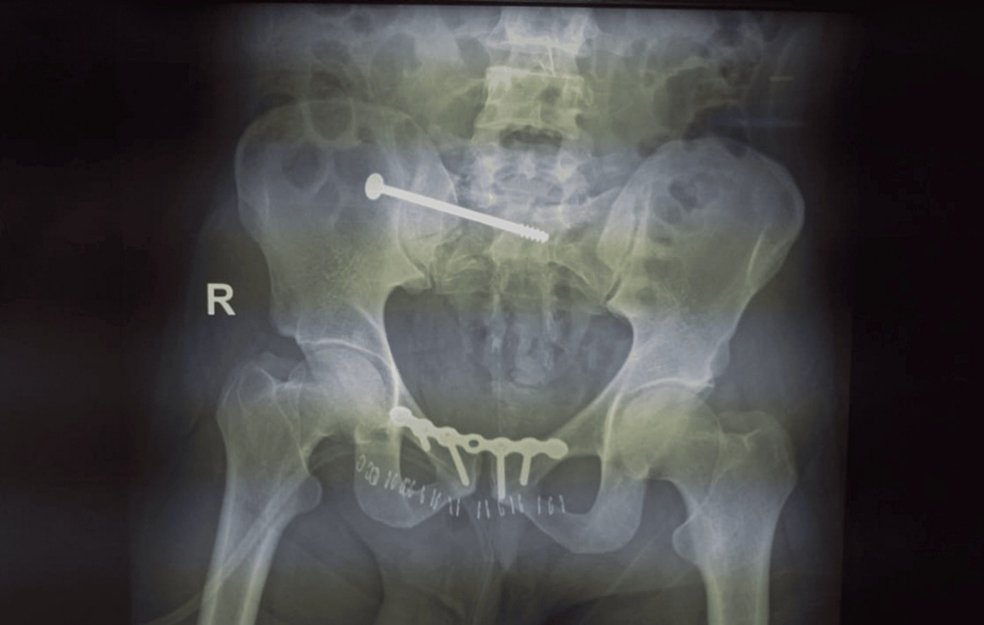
Postoperative X-ray posteroanterior view of the pelvis showing excellent reduction and fixation of the sacral fracture using an iliosacral screw with fixation of the anterior pelvic ring injury using anterior symphysis plating with the good restoration of ring alignment Image Credit: First Author
